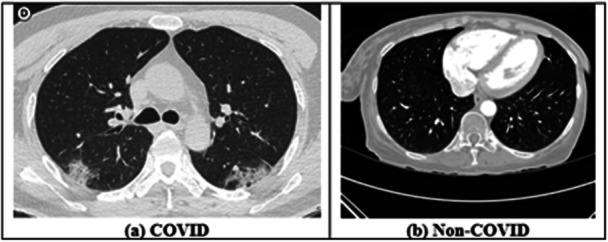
CT‐scan images from the dataset.
